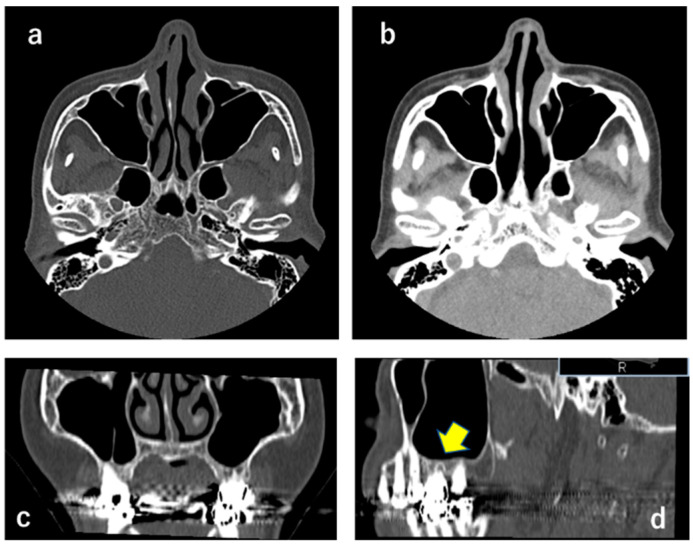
CT X-ray showing that masses were not observed in the left maxillary sinus (a–c). (b) indicates soft tissue density. A sagittal section ((d); yellow arrow) shows an apical periodontitis-like image at the maxillary left first molar, suggesting that this was not relevant to the clinical findings.
